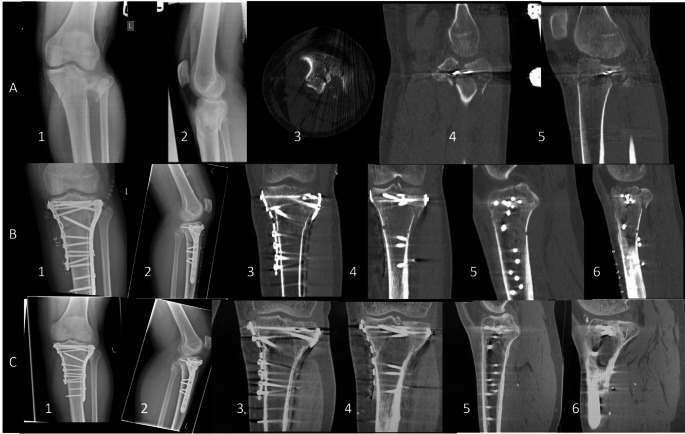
Male 27yoa, Schatzker VI or AO/ASIF 41C3 fracture with large lateral condyle joint depression (visible at A1 plain x-ray), managed initially with a bridging the knee external fixator and 2 percutaneous buried 1.6 mm k-wires (visible at A4 and A5 cuts) as means of temporary reduction/fixation till definite fixation. A dual surgical approach and plate fixation with joint surface rafting with screws and augmentation with the autologous bone grafting (anterior iliac crest) was performed 6 days later. Row A includes plain x-rays and CT scan slices on arrival: AP X-ray (A1); lateral x-ray (A2); axial cut at joint level (A3); coronal cut (A4); sagittal cut (A5). Row B includes plain x-rays and CT scan slices immediately postoperatively: AP x-ray (B1); lateral x-ray (B2); coronal cuts (B3 & 4); sagittal cuts (B5 & 6). Row C includes plain x-rays and CT scan slices at 12 months postoperatively: AP x-ray (C1); lateral x-ray (C2); coronal cuts (C3 & 4); sagittal cuts (C5 & 6). In comparison to immediate postop imaging there is evidence of complete healing and absence of secondary collapse at the comminuted lateral tibial plateau joint surface
